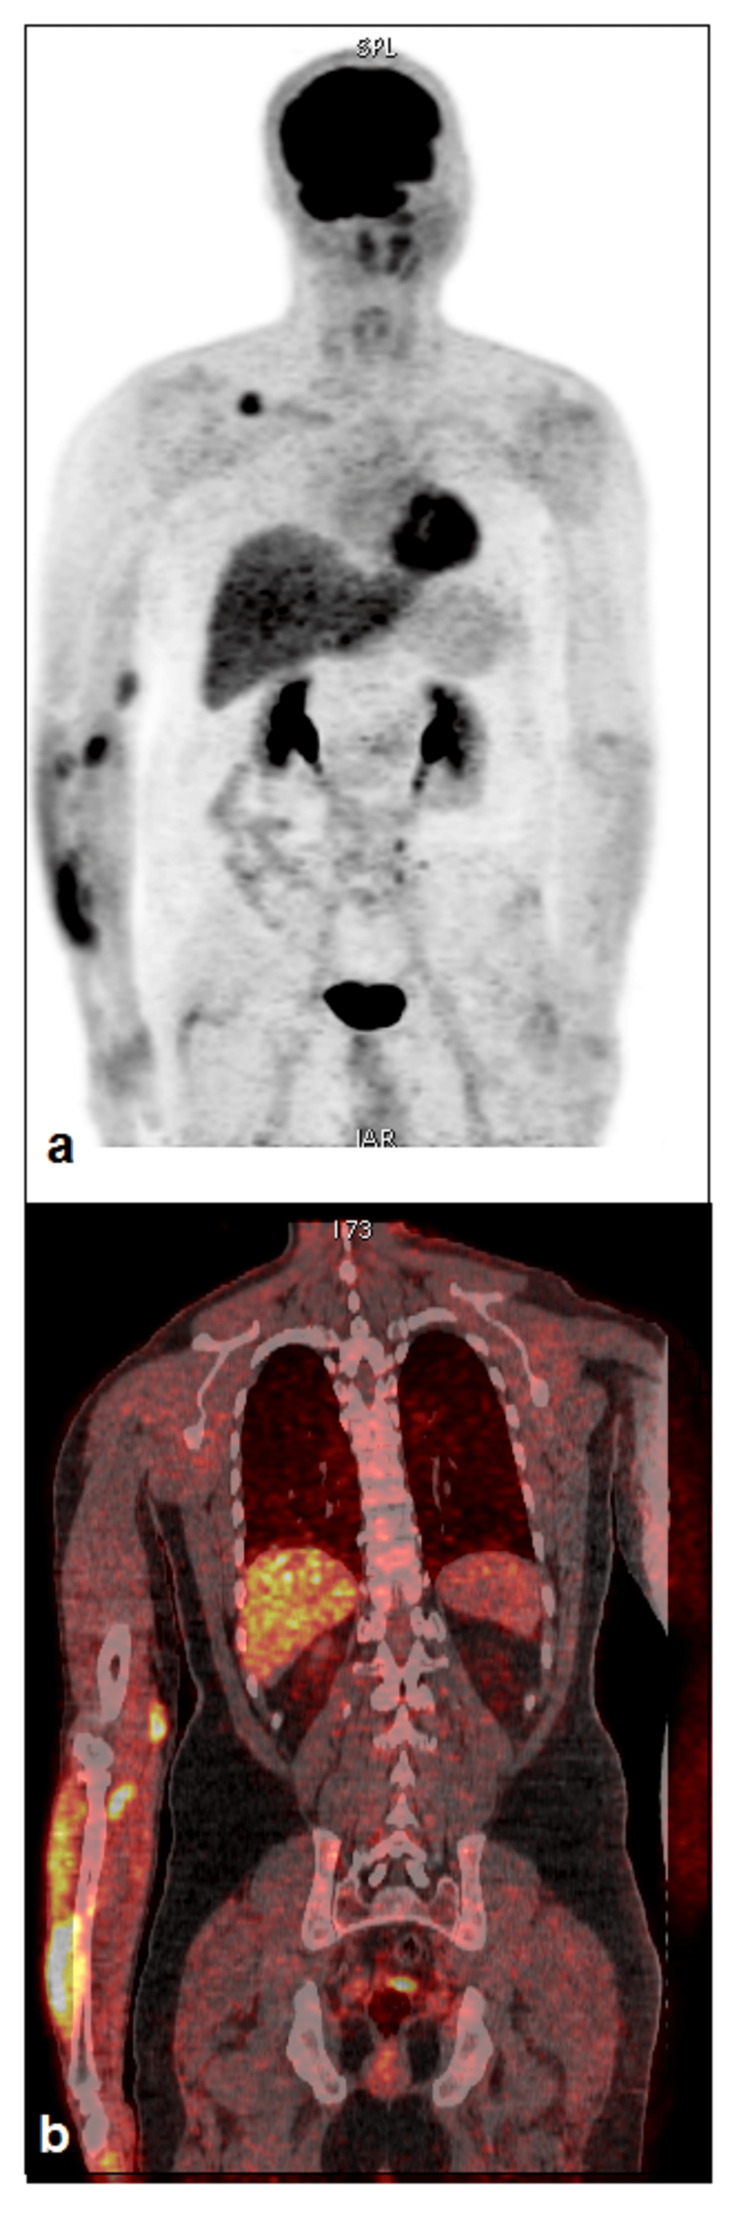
Maximum intensity projection (a) and coronal 18F-fluorodeoxyglucose (FDG) positron emission tomography/computed tomography fusion (b) showing FDG uptake throughout the soft tissues of the right upper limb and subclavicular region.
